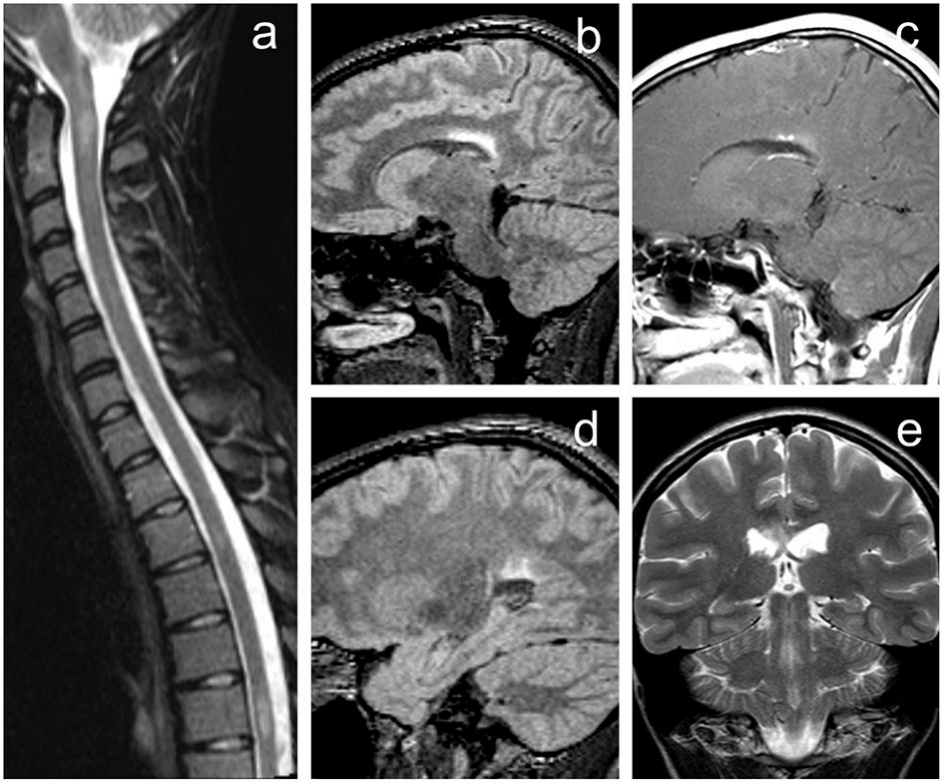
Initial MRI of the brain demonstrating rather typical finding of demyelinating disease fulfilling McDonald diagnostic criteria for multiple sclerosis. (a) Short-tau inversion recovery (STIR) image of the cervical and upper thoracic spine in sagittal plane revealing several hyperintense lesions (level C1/2, Th3/4, and Th6). (b,d) 3D fluid-attenuated inversion recovery (FLAIR) images of the brain in the sagittal plane. (e) T2-weighted image in the coronal plane. (c) Contrast-enhanced T1-weighted image with magnetization transfer in sagittal plane. Brain MRI revealed several periventricular white matter lesions including the largest FLAIR hyperintense plaque within the corpus callosum (b,e) with punctate enhancement (c). Image (d) shows some of the other smaller lesions near the trigone of the left lateral ventricle.
